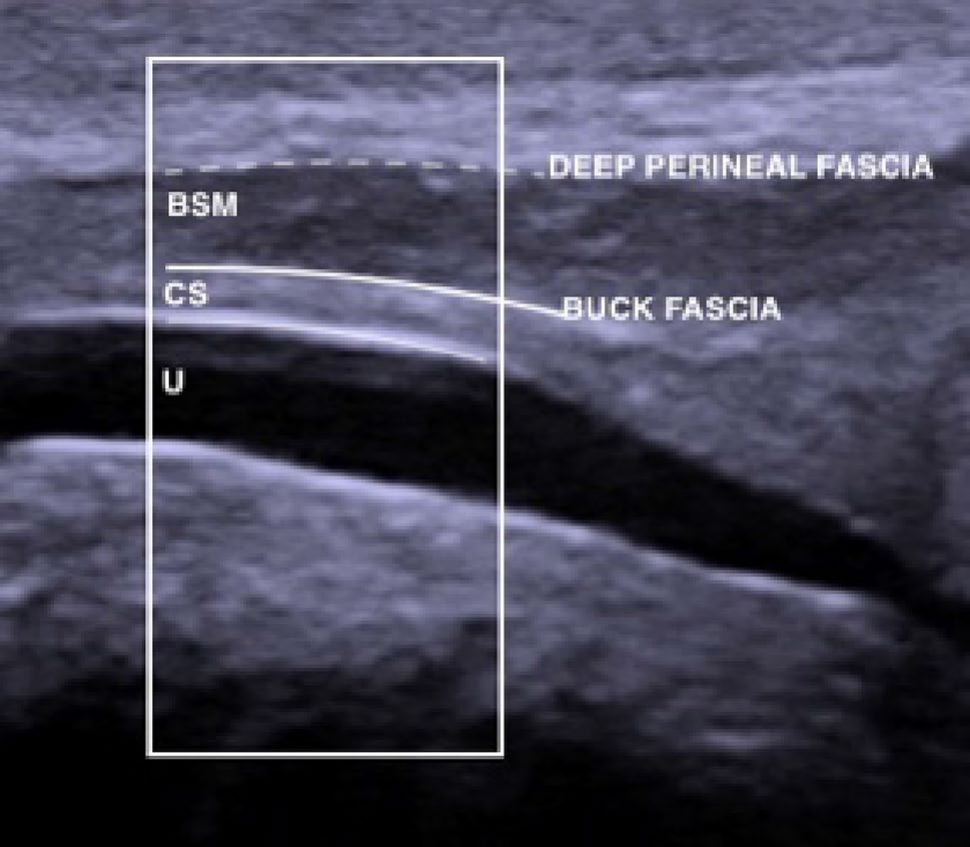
Ultrasound image of bulbar urethra in longitudinal scan shown within the white box (Figure provided by the authors) *U* urethra. *CS* corpus spongiosum. *BSM* bulbospongiosus muscle. Thin white line—urethral epithelium, thick white line —Buck’s fascia, dotted line— *DPF* deep perineal fascia
